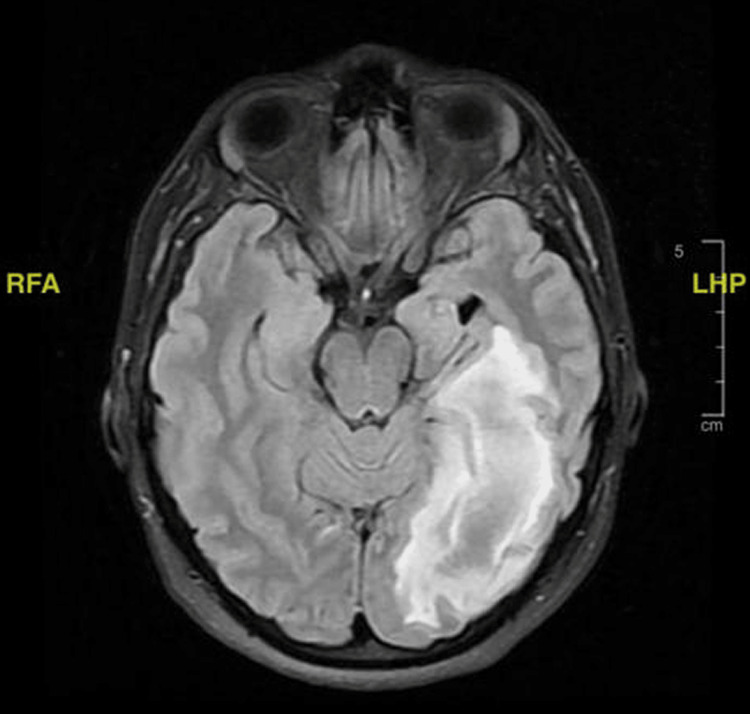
A FLAIR sequence MRI in axial view showing a large lesion of low T1 signal measuring approximately 6.3 × 3.4 × 2.4 cm in AP, TX, and CC dimensions, respectively. Additionally, surrounding vasogenic edema can be noted at the left occipital lobe and the posterior aspect of the temporal lobe. The initial picture is suggestive of hyperacute hematoma due to the onset, and an abscess or necrotic hemorrhage or tumor is less likely. FLAIR, fluid-attenuated inversion recovery; MRI, magnetic resonance imaging; AP, anteroposterior; TX, transaxial; CC, craniocaudal; LHP, left, head, posterior; RFA, right, foot, anterior
